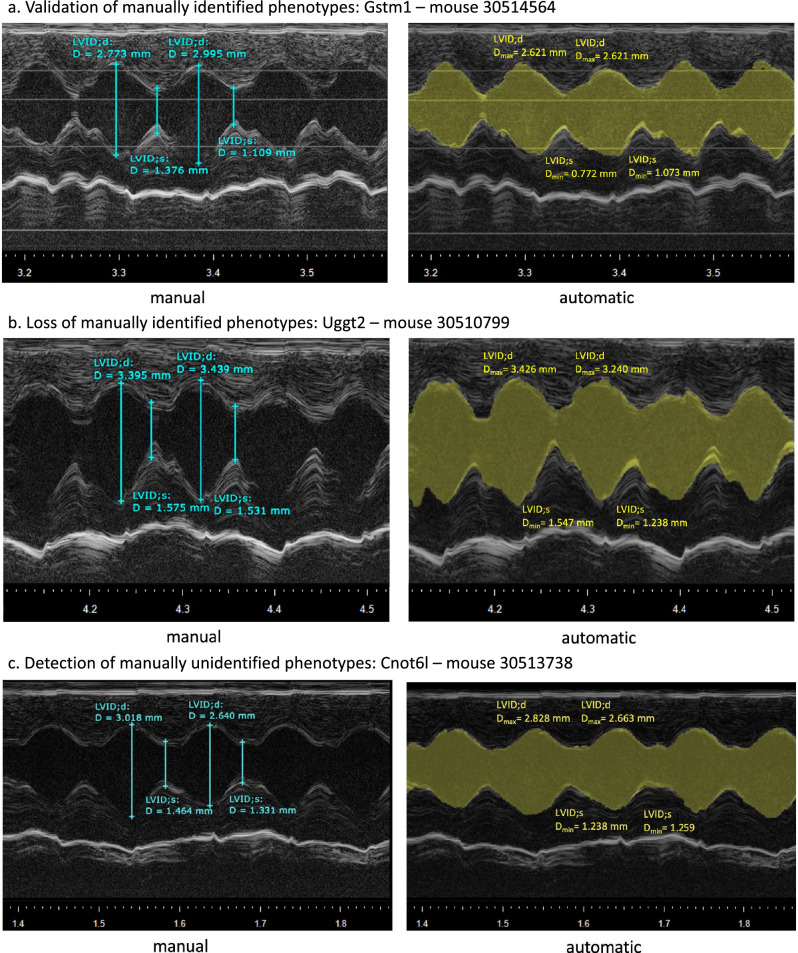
Representative echocardiograms (Visual Sonics, Vevo2100, short axis, M-mode) for comparison of manually and automatically extracted features. Manual annotation shown in the left panel is based on only two consecutive heartbeats whereas automatic Echo2Pheno-based annotation is considering the entire dataset. Panel a Validation of manually identified phenotypes by an agreement of manual (left panel) and automatic (right panel) analysis in *Gstm1*; Panel b Loss of manually identified phenotypes in LVIDs, EF, and FS due to a mismatch between manual (left) and automatic (right) analysis in *Uggt2*; Panel c Detection of manually unidentified phenotypes in *Cnot6l* (LVIDs for both sexes) not found with the manual annotations
